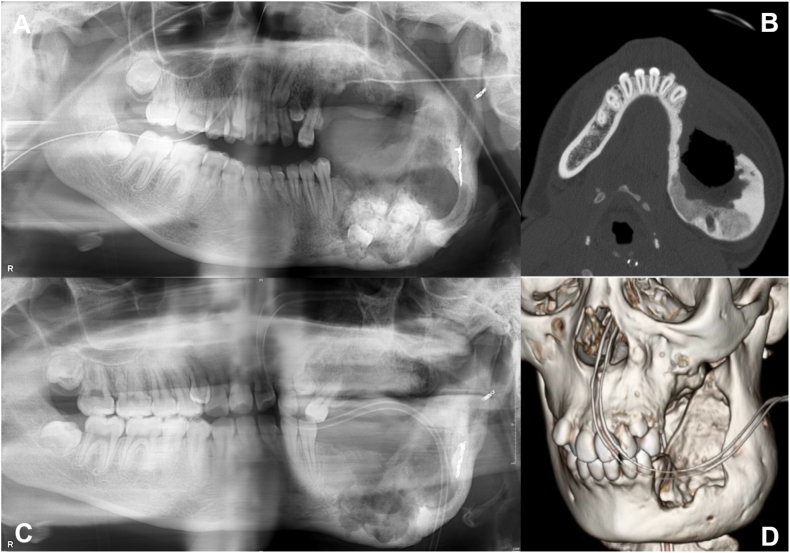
Postoperative findings. A: Panoramic radiography showing 2/3 of the odontoma was removed after the 1st surgery. B: Horizontal computed tomography image showing good bone formation in the mandible 3 months after the 1st surgery. C,D: Panoramic radiography and 3-dimensional computed tomography after 2nd tumor removal surgery showing complete removal of the mandibular odontoma.
